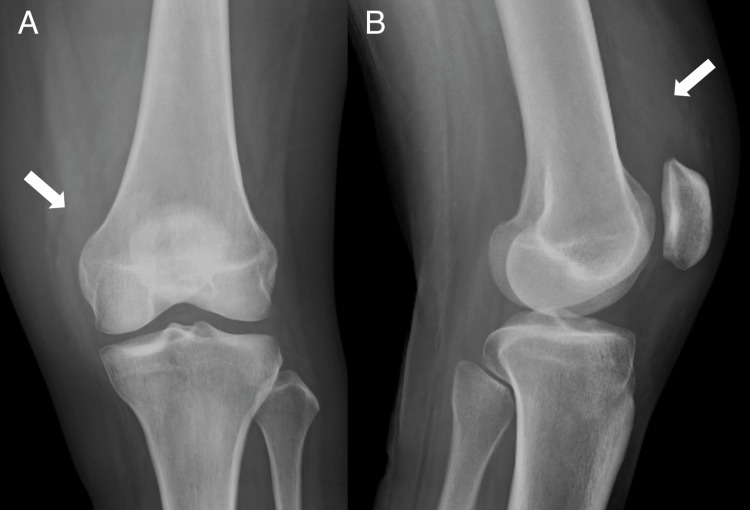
Left knee anteroposterior (A) and lateral (B) X-ray. Immature heterotopic ossification (HO) is identified in the medial knee (arrows). Immature heterotopic ossification in the medial knee (arrows).
